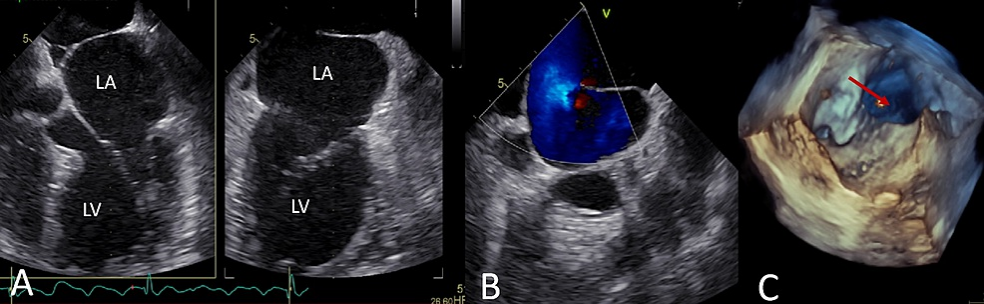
Transesophageal echocardiography characterizing the cor triatriatum sinistrum. The LA is partially divided by a nonrestrictive membrane, shaped like a diaphragm, with a single posterior wide opening (red arrow). A) Biplanar mid-esophageal view; B) modified mid-esophageal view at 90º; C) Three-dimensional reconstruction of the septum, atrial view.
